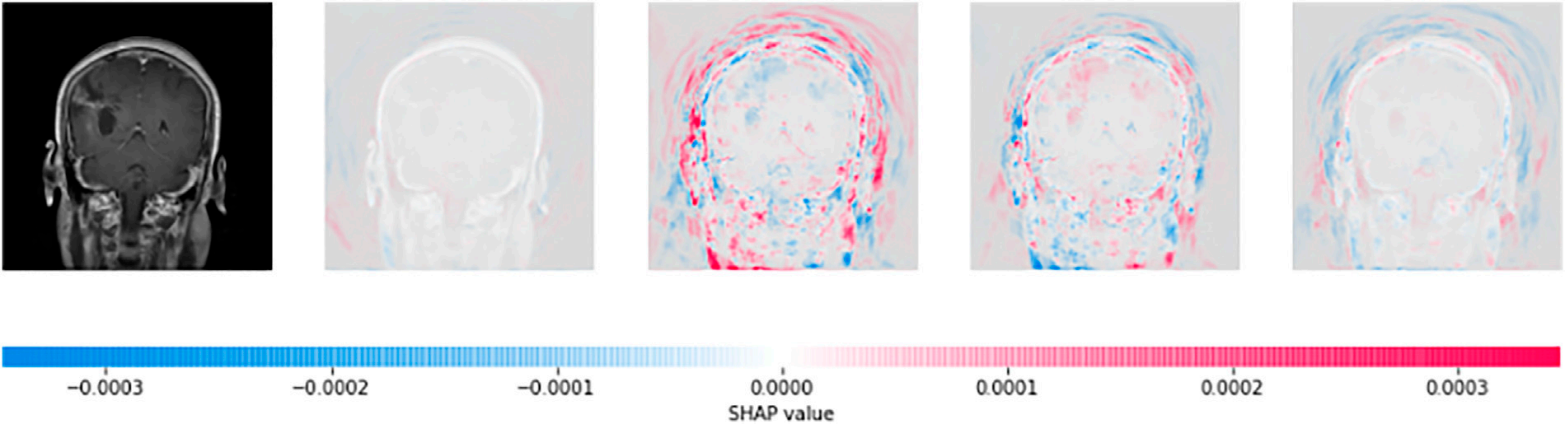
On the basis of Shapley values, we can say that the MRI image holds meningioma tumour.
